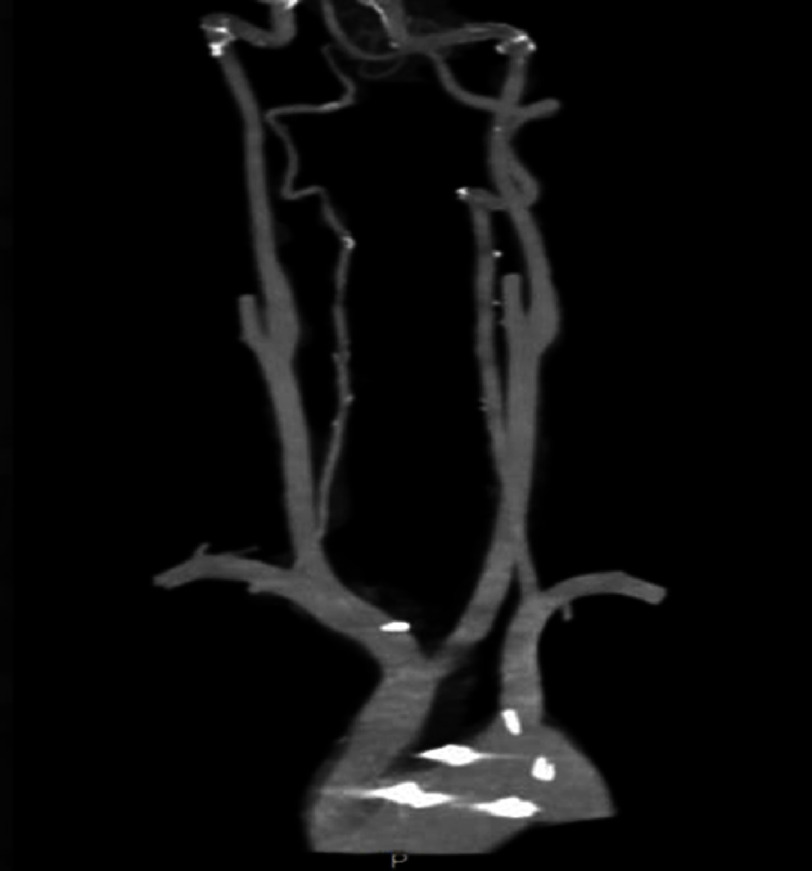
CTA of head and neck, demonstrating patent carotid and vertebral arteries, without any vascular malformation, thickening, aneurysm or stenosis.
